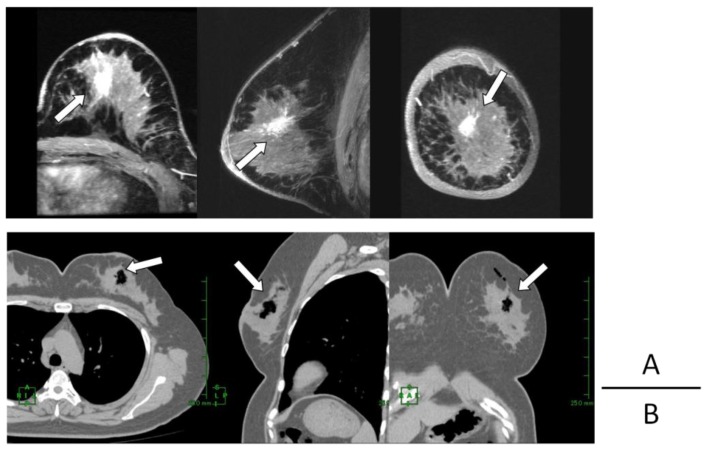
Case 6: Comparison of the position of tumor (arrows in A) and of generated oxygen gas (arrows in B) (A) 3D-MIP images of pre-treatment CE breast MRI (B) 2D reconstructed breast CT images taken approximately 3 h after KORTUC radiosensitization agent injection.
